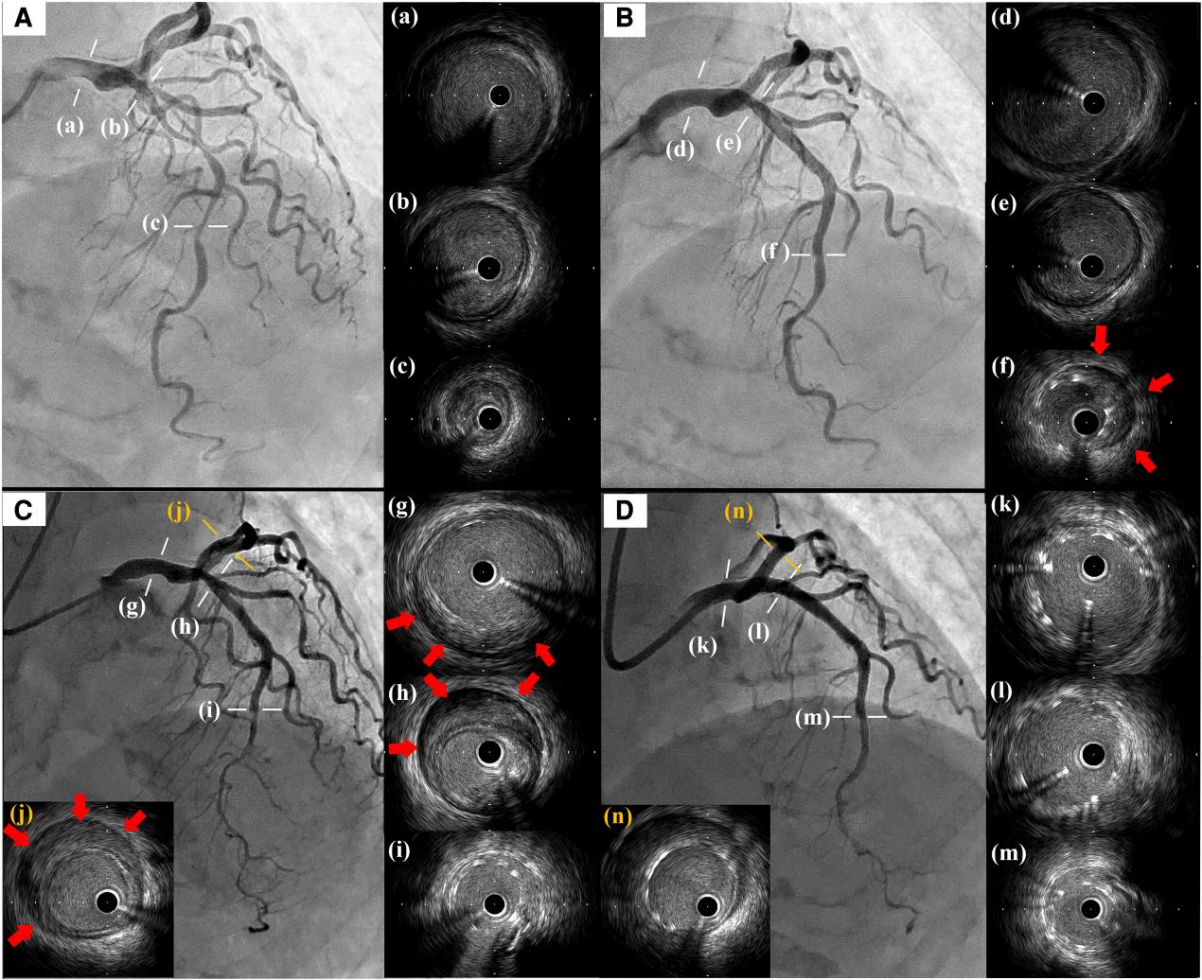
(*A*) Initial left coronary angiography from a straight cranial view and images of IVUS (a–c). IVUS demonstrated no haematoma but low-echoic plaque. (*B*) Post-primary PCI. IVUS revealed haematoma in the stent distal (red arrows) but not in the proximal vessel (d–f). (*C*) Emergent coronary angiography and IVUS on day seven. The previous haematoma extended to the LMT through the LAD (g–i) and even to the proximal LCX (j). Red arrows indicated the newly observed haematoma. (*D*) Final angiography and IVUS after the bailout PCI. Additional stents were implanted, covering the LMT to the LAD (k–m) and the LCX (n) using the T-stent methods. IVUS, intravascular ultrasound study; PCI, percutaneous coronary intervention; LMT, left main trunk; LAD, left anterior descending artery; LCX, left circumflex artery.
